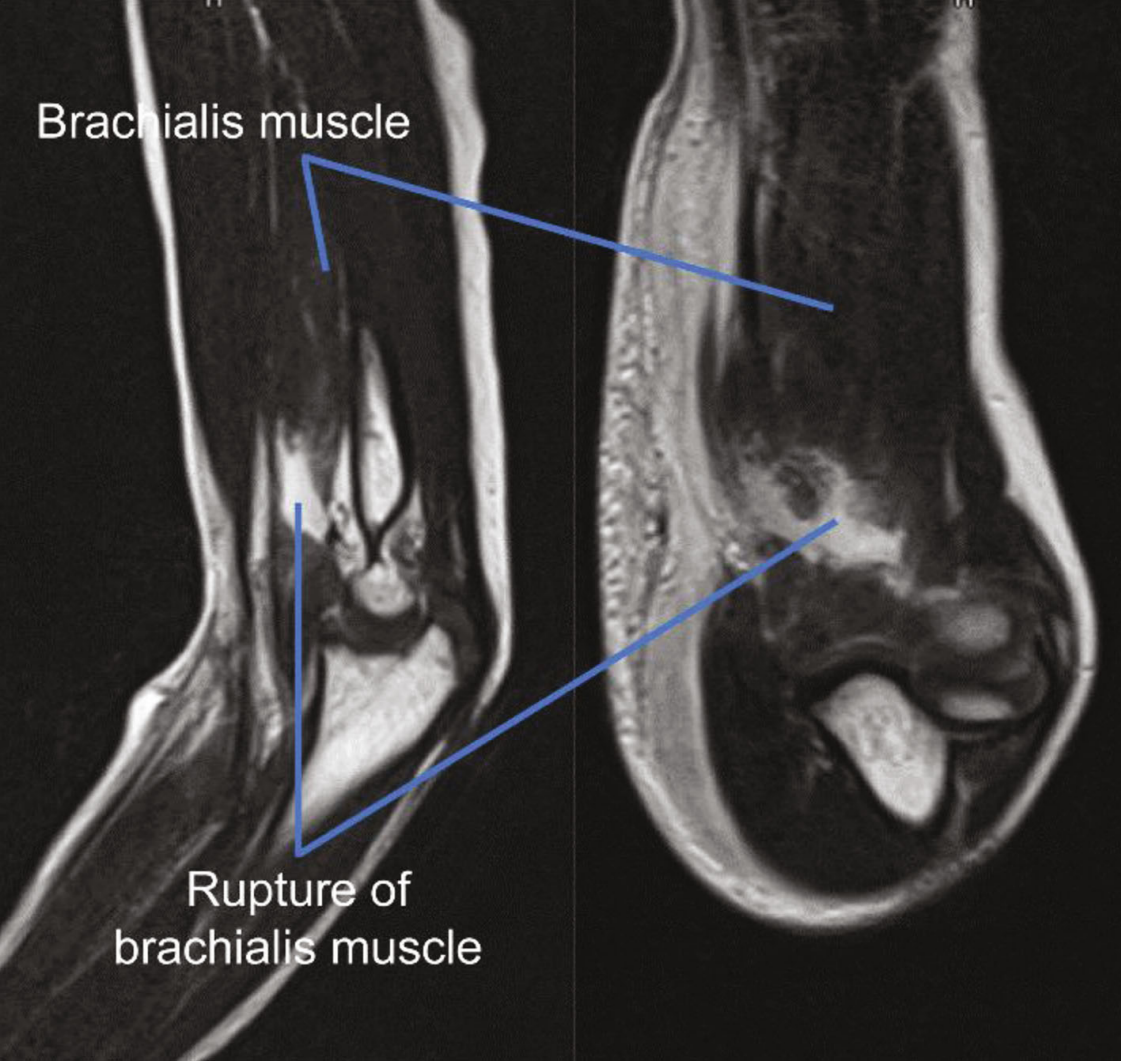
T2 magnetic resonance imaging.
